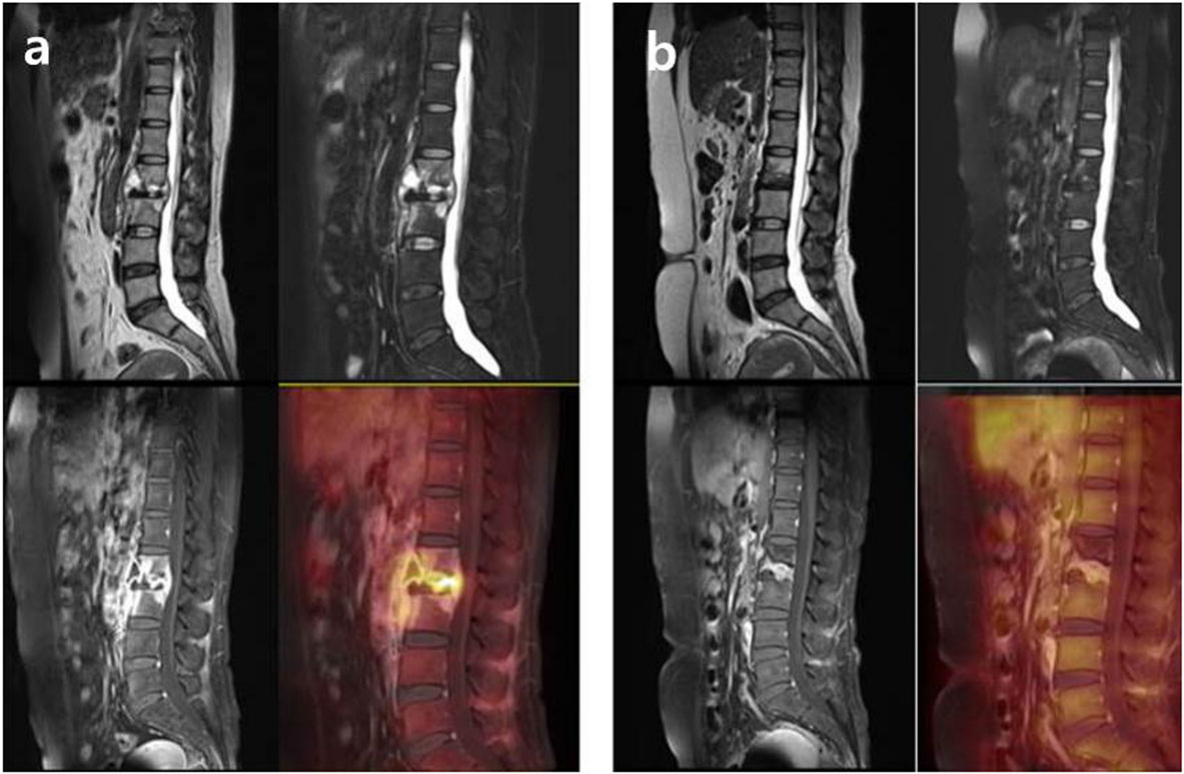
The first patient’s PET/MRI at 12-month anti-TB medication therapy (b) revealed the decreased bone marrow edema, pre- and intra-vertebral abscesses, paraspinal soft tissues, and SUV_max_ (from 9.75 to 1.83) on L2–3 compared with PET/MRI at 4-month anti-TB medication therapy (a). There was no relapse during the one-year follow-up period after discontinuation of treatment
